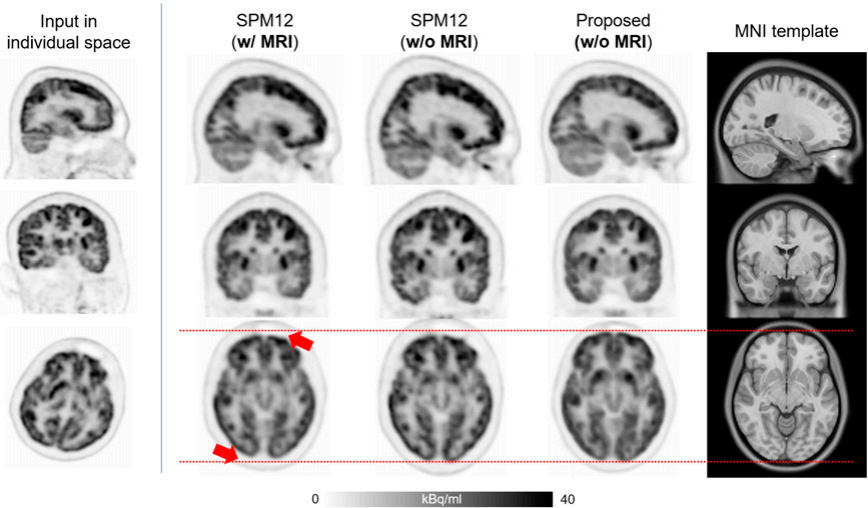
^18^F-FDG PET images of 13-y-old boy with epilepsy, included in internal dataset. Small brain in individual space was not adequately resized by SPM, as indicated by red arrows.
